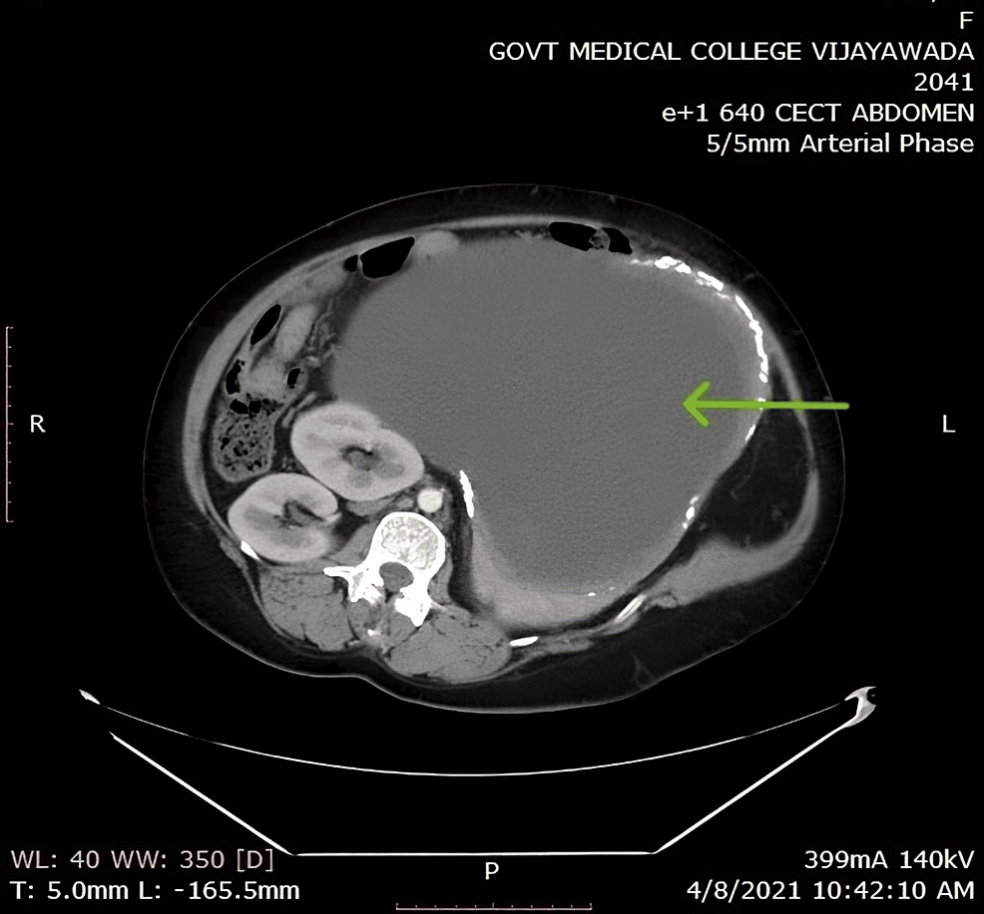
A CECT abdomen of the patients showing the splenic hydatid Axial section of a contrast-enhanced CT scan of the abdomen (green arrow pointing the splenic hydatid)
